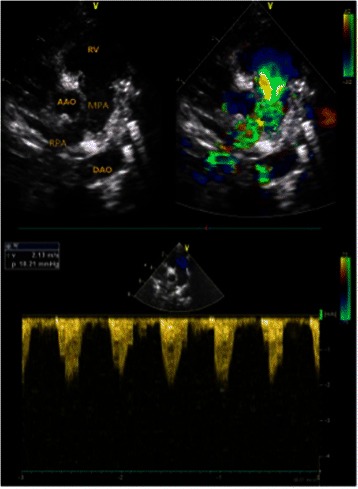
Postoperative echocardiography of Patient 6
